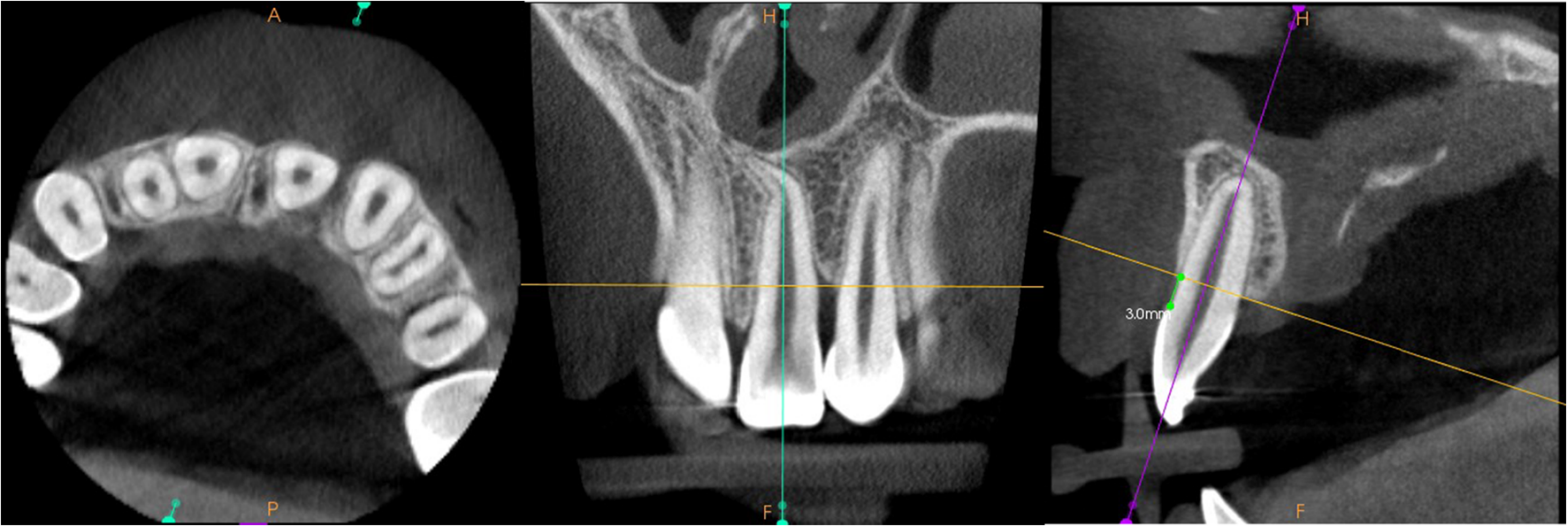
The CBCT images standardization
